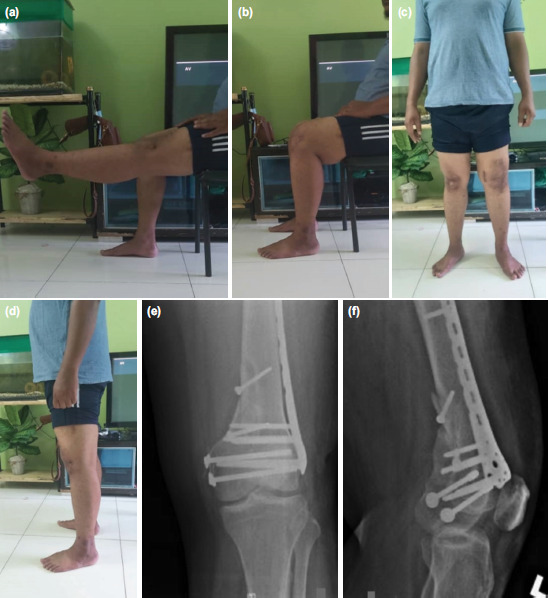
(a, b) Clinical picture showed the patient was able to flex full extension and his left knee up to 90°, (c, d) the patient was able to stand without any aids, (e, f) post-operative radiograph AP and lateral view over left distal femur showed fracture have united.
